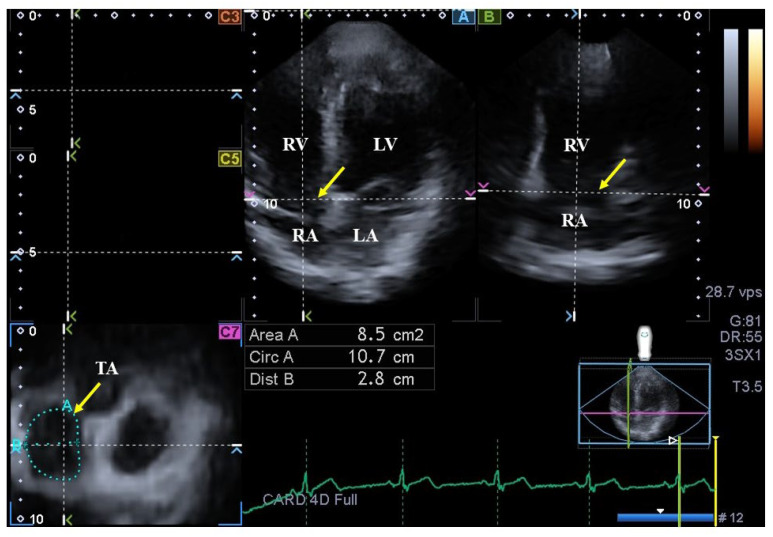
Assessment of the tricuspid annulus (TA) is presented extracted from a three-dimensional echocardiographic full-volume dataset: apical four-chamber view (A); apical two-chamber view (B); and a cross-sectional view at the level of the TA optimized in apical four- and two-chamber views (C7); short-axis views at basal (C3), midatrial (C5). The yellow arrow represents the tricuspid annular plane. Abbreviations: LA = left atrium, LV = left ventricle, TA = tricuspid annulus, RA = right atrium, RV = right ventricle, Area = TA area, Circ = TA perimeter, Dist = TA diameter.
